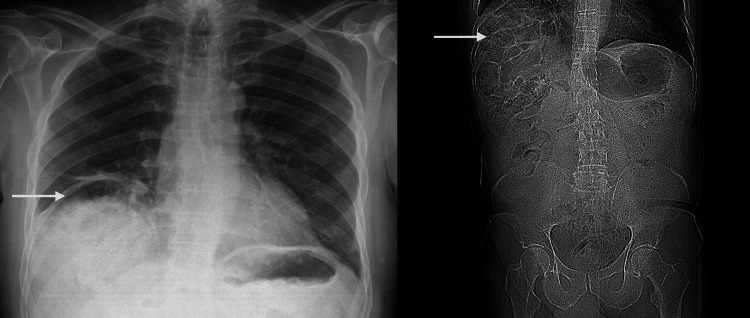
Chest X-ray and the scout image of the abdomen and pelvis CT, A gas-filled loop of colon is interposed between the liver and the right hemidiaphragm, mimicking free intraperitoneal air.
